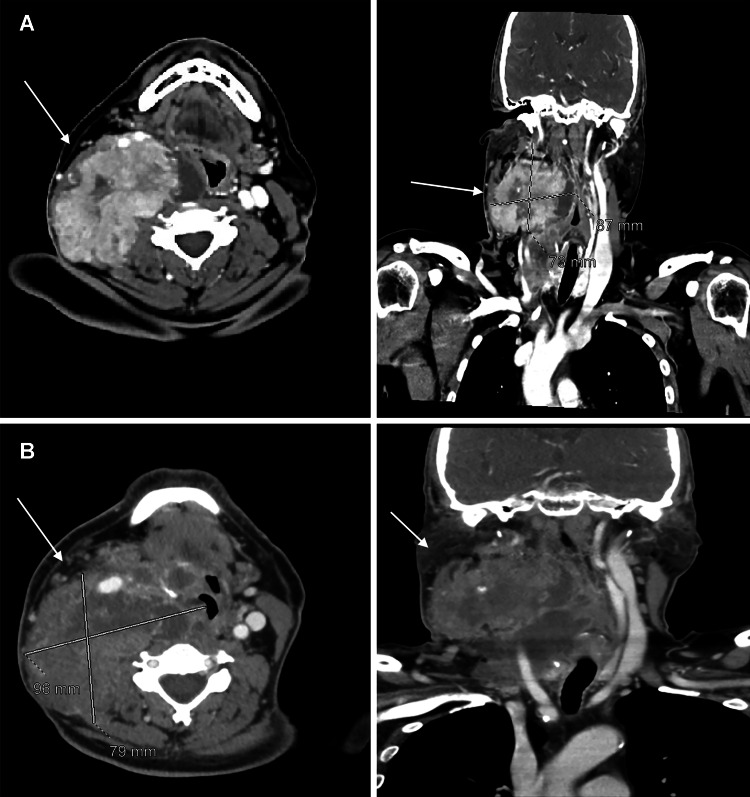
A) Neck CT with axial and coronal views prior to the evaluation with WBS and rhTSH. B) Neck CT with axial and coronal views one and a half months after using rhTSH, demonstrating an increase in the size of both lesions, primarily in the thyroid CT: computed tomography; rhTSH: recombinant human thyroid stimulating hormone; WBS: whole-body scan
